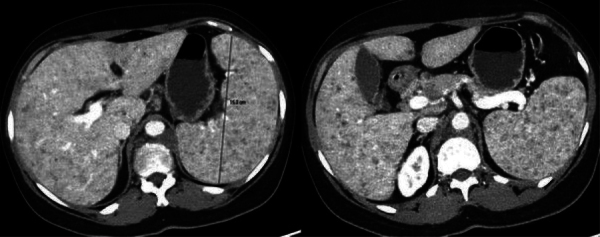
Axial contrast-enhanced CT
